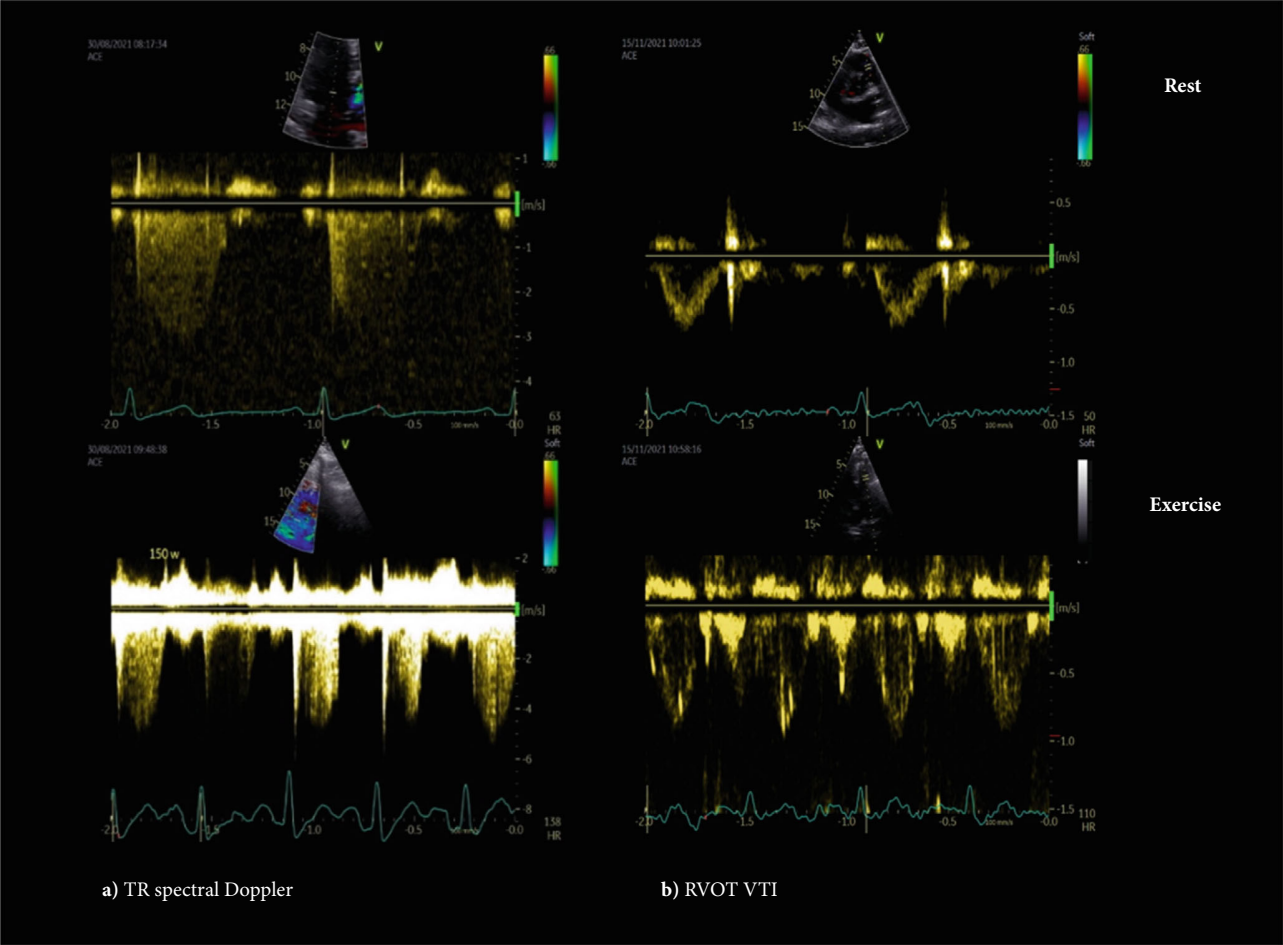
Echocardiographic variables for assessing the mean pulmonary artery pressure/cardiac output (mPAP/CO) slope in two patients. (a) Tricuspid regurgitation (TR) spectral Doppler envelopes demonstrating signal extension throughout systole at rest and with the injection of suspended bubbles in 0.9% NaCl during exercise. (b) Right‐ventricle outflow tract velocity–time integral (RVOT VTI) by Doppler at rest and during exercise.
